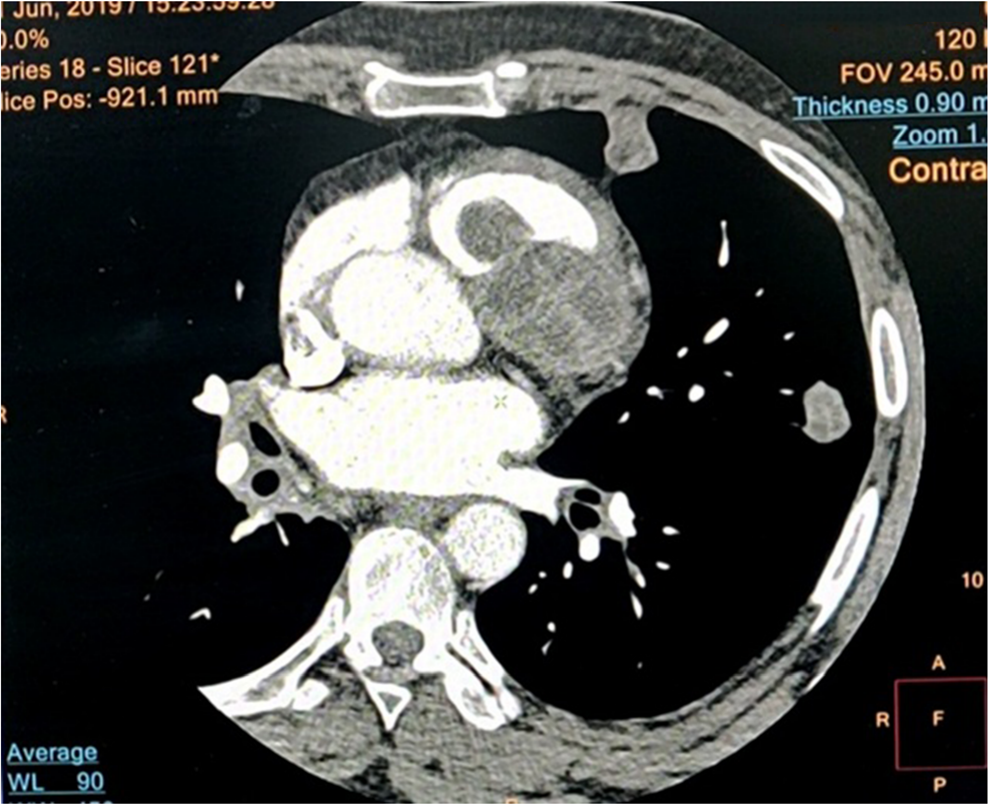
CT of the thorax showing multiloculated cystic lesion in the right ventricular outflow tract and multiple lung lesions
